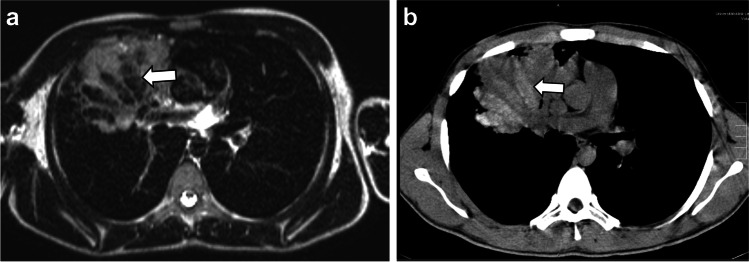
A 16-year-old boy with cystic fibrosis. A T2 fast spin echo magnetic resonance (MR) image (a). The mucus in the bronchiectasis in the basal right upper lobe is hypointense on T2 (*arrow*). An axial unenhanced computed tomography (CT) image (b). The mucus in the bronchiectasis in the basal right upper lobe is hyperdense on CT (*arrow*). This combination of MR and CT findings is characteristic of allergic bronchopulmonary aspergillosis and aspergilloma
